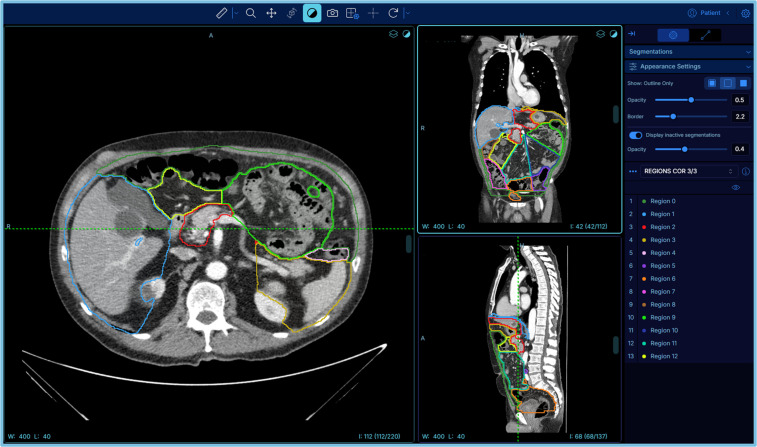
Screenshot of multiplanar reconstructions of a CT scan during evaluation with pre-segmented PCI regions, visualized using coloured boundaries. The image includes a screenshot of the OHIF Viewer interface, reproduced under the MIT License (Copyright © 2018 Open Health Imaging Foundation).
